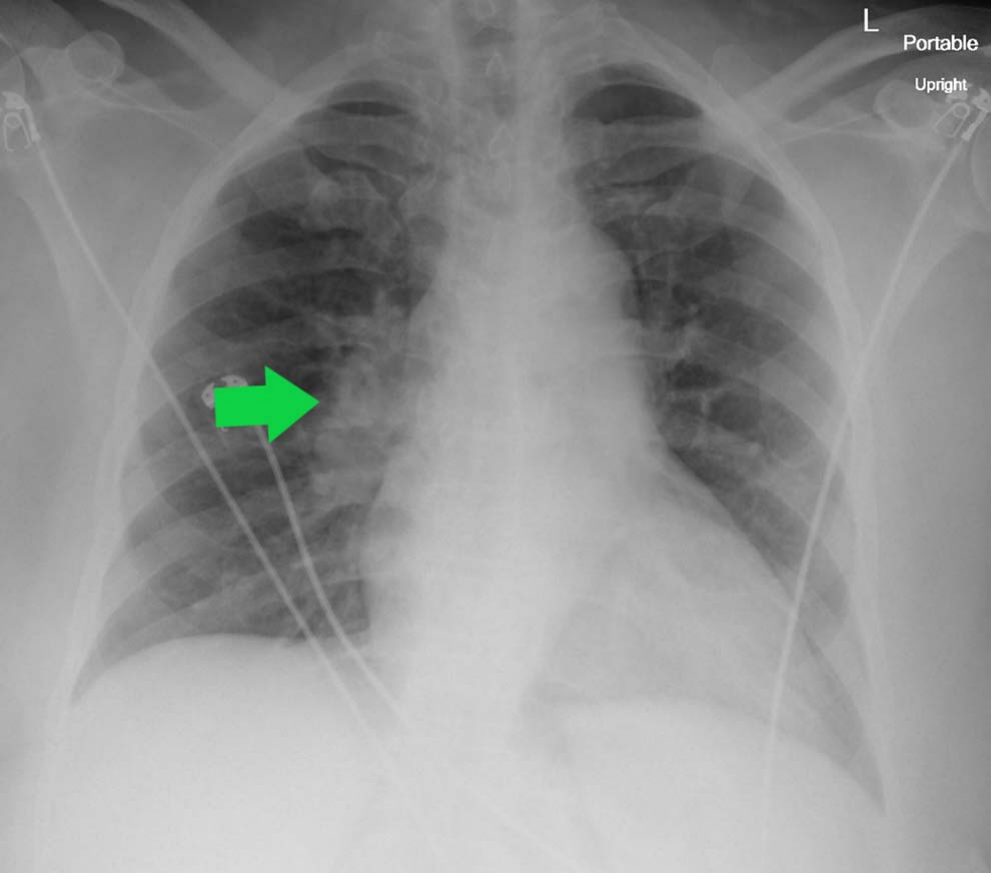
Portable upright anteroposterior radiograph of a 68-year-old female shows prominence of the right hilar shadow (arrow) and otherwise clear lungs. This pattern was considered atypical and less suspicious for COVID-19.
